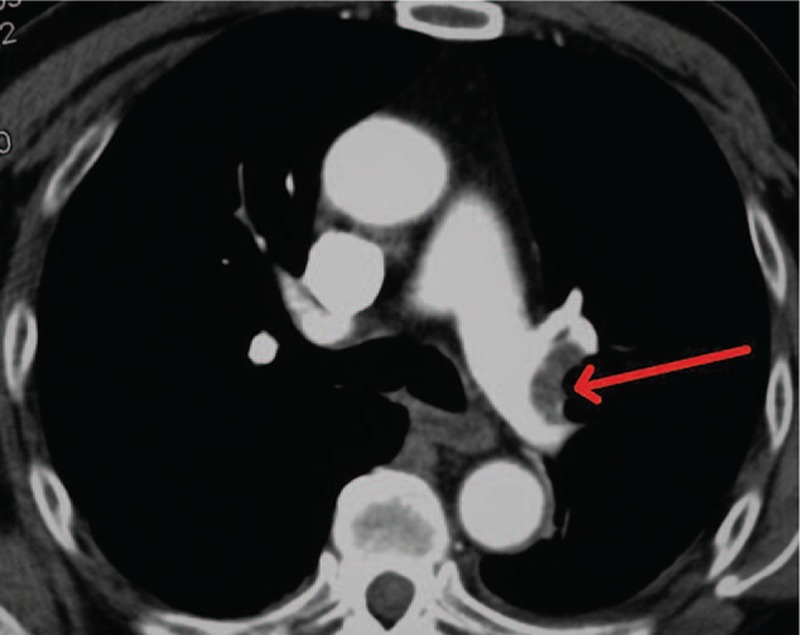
CTPA confirmed the diagnosis of PTE in the left superior and left inferior pulmonary arteries in case 2. CTPA = computed tomographic pulmonary angiography, PTE = pulmonary thromboembolism.
